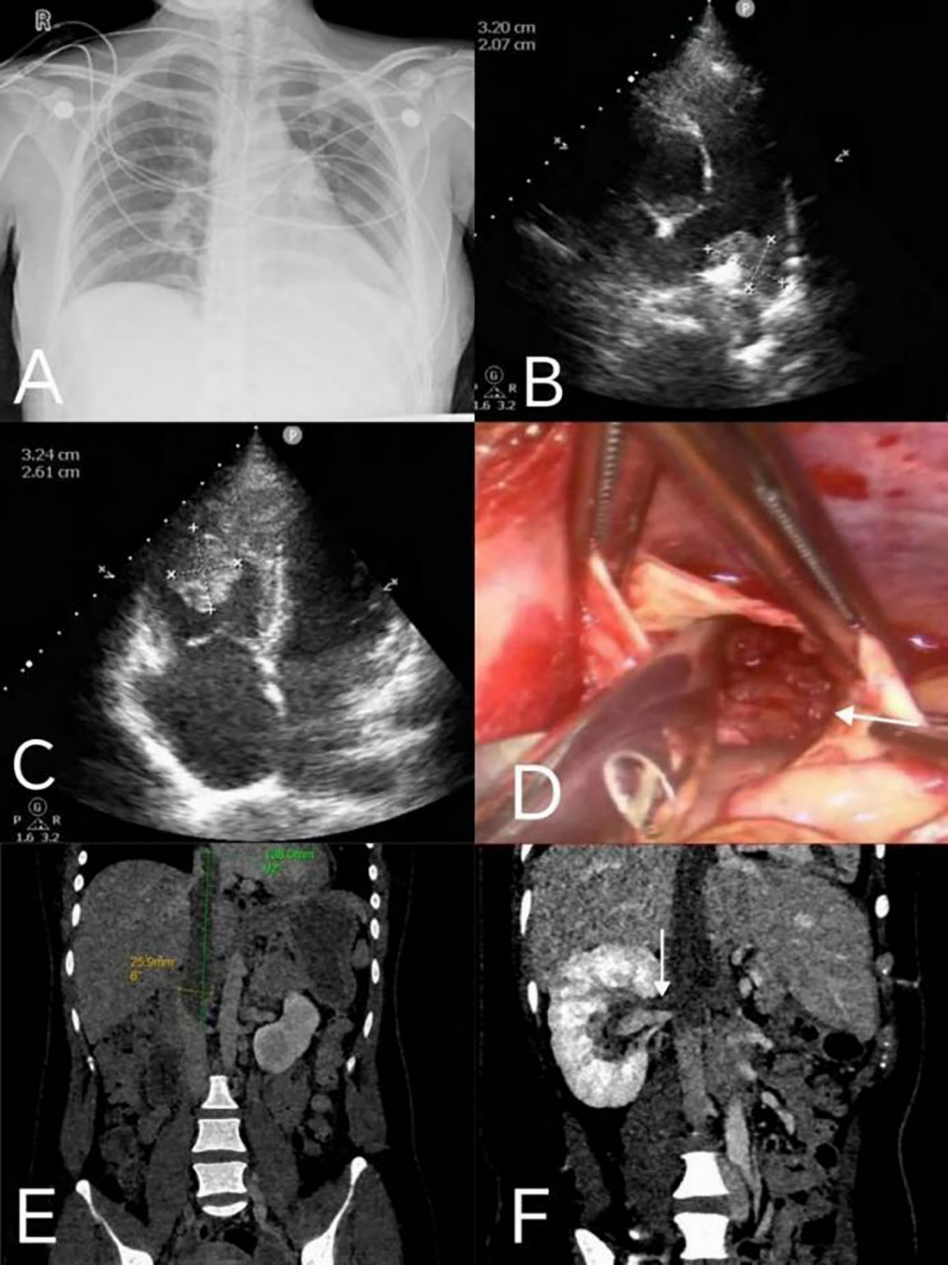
The results of imaging examination. (A) Chest radiography when first admission (B) Echocardiography revealed thrombosis of the pulmonary artery when first admission (C) Echocardiography revealed thrombosis in the right ventricle when first admission (D) Thoracoscopic intracardiac and pulmonary thrombus removal using extracorporeal circulation (E) Main abdominal vein computed tomography angiography shows that embolism in the cava when third admission (F) Main abdominal vein computed tomography angiography shows that embolism in the renal veins when third admission
